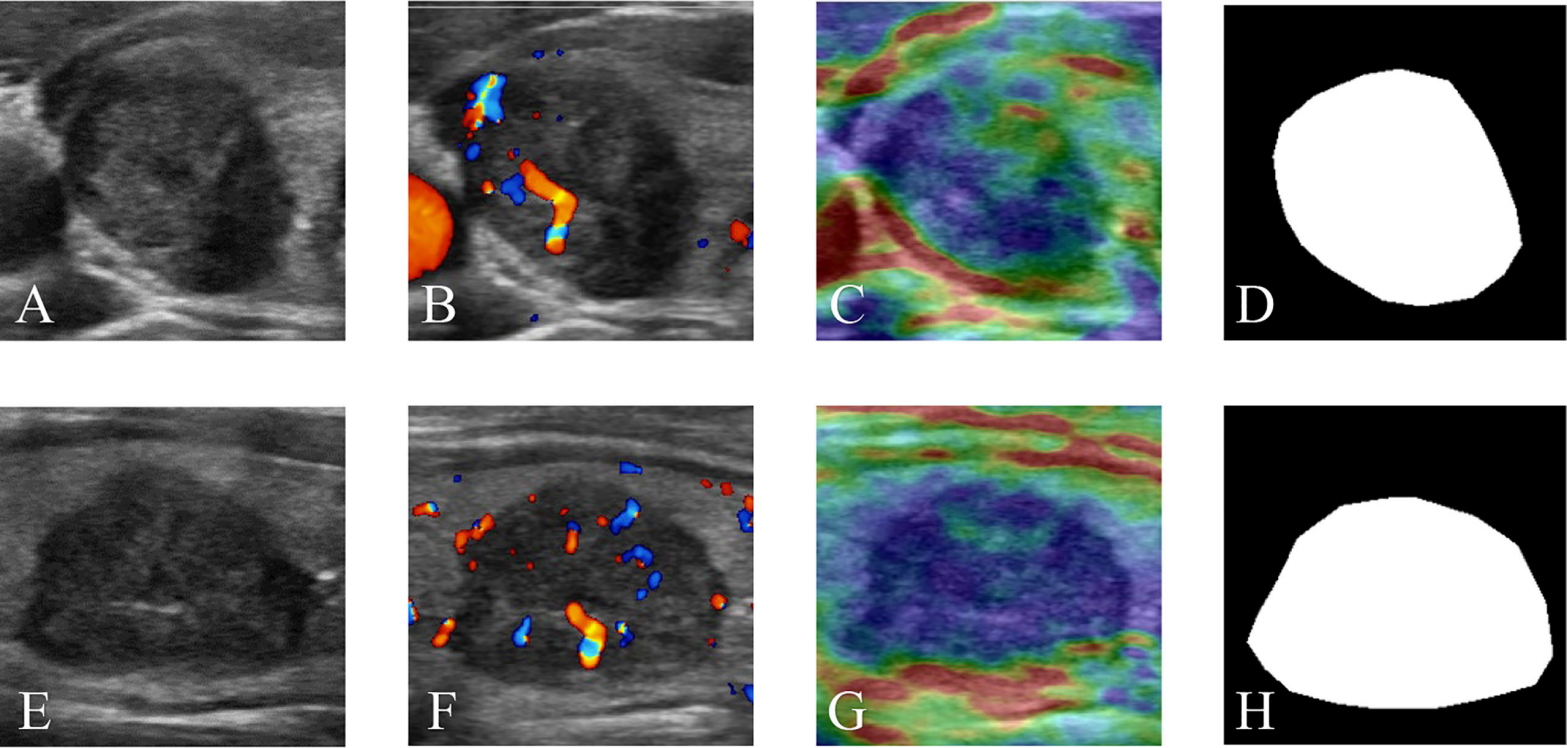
Multimodal ultrasound images of a thyroid nodule in the right lobe of a 65-year-old female patient with a pathologically proven papillary carcinoma. (A) GSU, (B) CDFI, (C) SE, and (D) Mask ultrasound images in transverse section. (E) GSU, (F) CDFI, (G) SE, and (H) Mask ultrasound images in longitudinal section. GSU, grayscale ultrasound; CDFI, color Doppler flow imaging; SE, strain elastography; Mask, region of interest mask.
